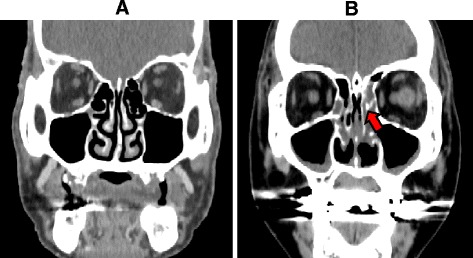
CT images of a normal 75-year-old man (a) and a 45-year-old woman diagnosed with ECRS (b). The *arrow* indicates opacity in the ethmoid sinuses
